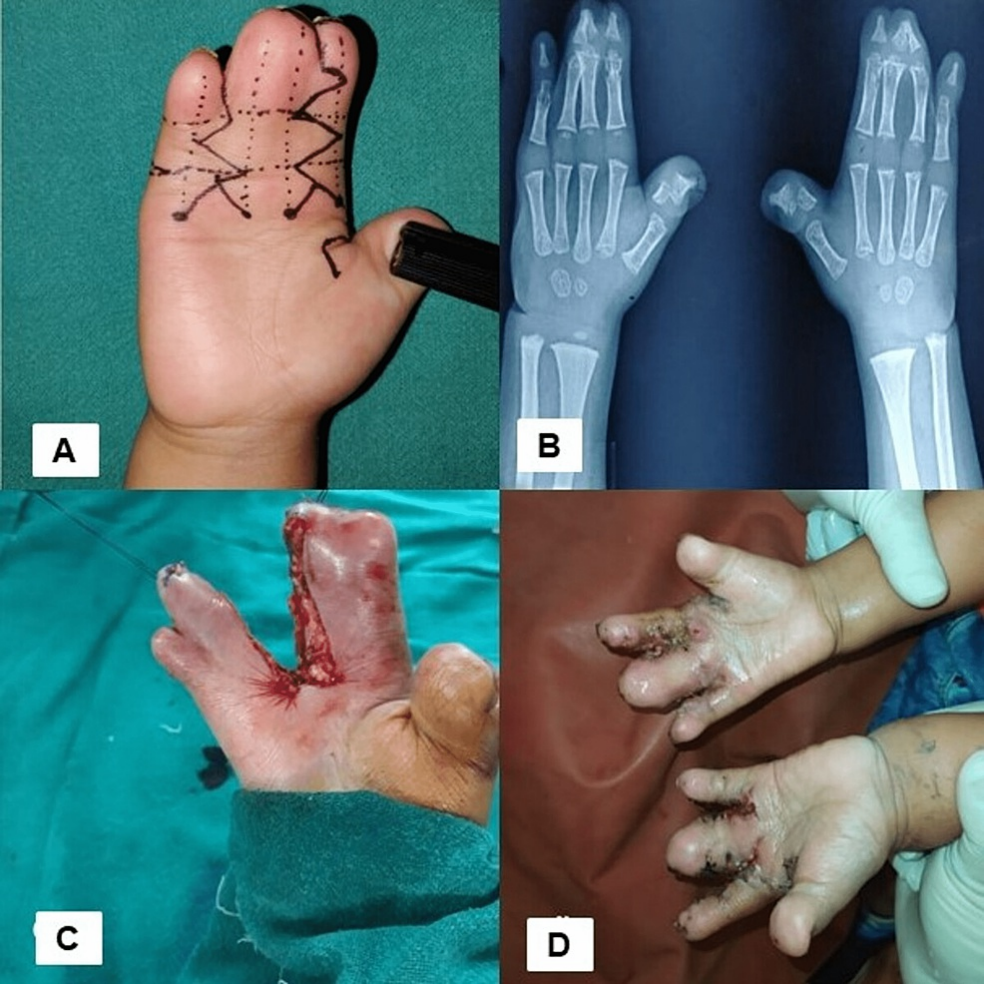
Images showing (a) planning and release of second web space complete syndactyly and 4th webspace incomplete syndactyly. (b) X-ray showing fusion of third and fourth distal phalanx in syndactyly. (c) Intraoperative after separation of digits. (d) Postoperative follow-up case.
